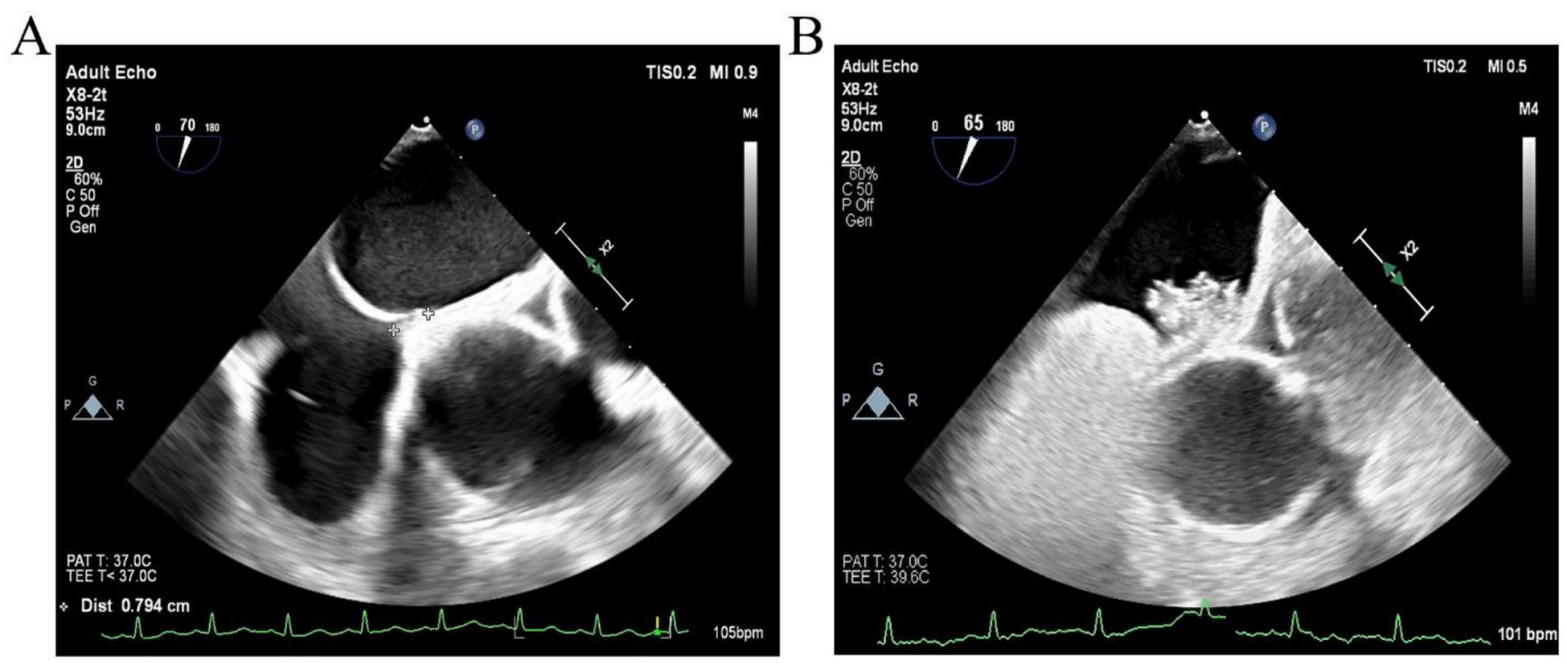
Transesophageal echocardiography (c-TEE) findings. (A) Measurement of the PFO tunnel length during Valsalva maneuver. (B) Real-time visualization of microbubble passage from the right to left atrium through the foramen ovale, confirming the presence of a right-to-left shunt (RLS).
